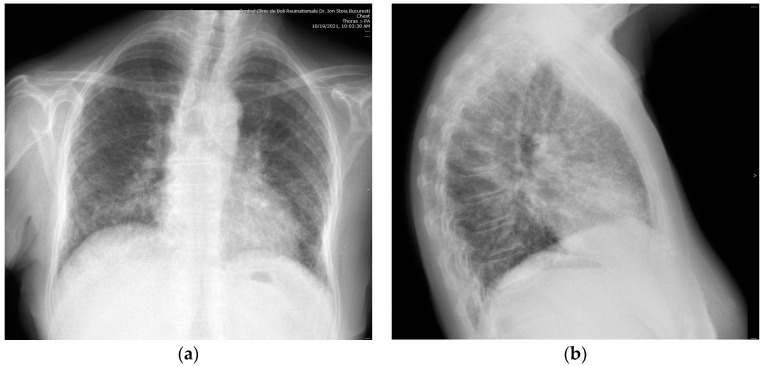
Radiographic ILD in a 71-year-old woman with seropositive RA showing diffuse interstitial fibrosis with bilateral reticular and areolar pattern (“honeycomb”) on (a) anteroposterior view and on (b) left lateral view.
